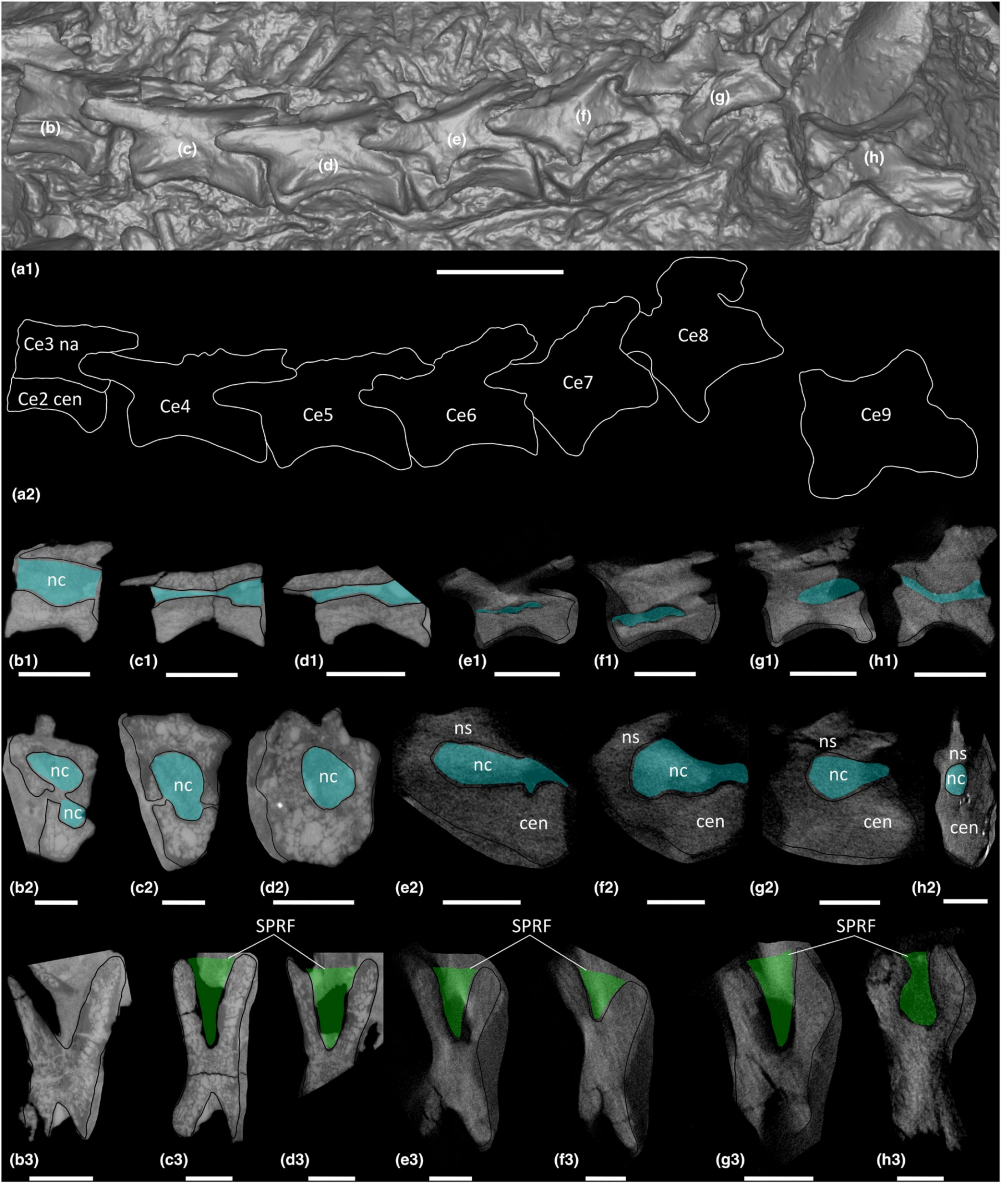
CT scan data of *Pantydraco caducus* cervical vertebrae. (a) lateral view of sequence (a1) surface scan, (a2) outlined; (b) cervical centrum 2 and cervical neural arch 3, (c) cervical vertebra 4, (d) cervical vertebra 5, (e) cervical vertebra 6, (f) cervical vertebra 7, (g) cervical vertebra 8, (h) cervical vertebra 9; (b1–h1) parasagittal cross‐section, (b2–h2) transverse cross‐section, (b3–h3) horizontal cross‐section. Ce, cervical vertebra; cen, centrum; nc, neural canal. Key: Blue highlight, neural canal; green highlight, fossa. Scale bars = 2 cm (a), 1 cm (b1–h1), 0.5 cm (b2–h3).
